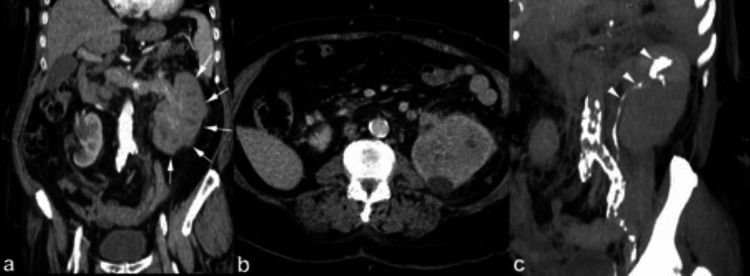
The contrast-enhanced CT (CECT) images during the arterial (a), nephrographic (b), and urographic (c) phases demonstrate the faceless kidney, which appears enlarged and displays a high density of soft tissue. Furthermore, the enhancement of the renal vein is modest due to tumoral invasion accompanied by indications of thrombosis. Large infiltrating transitional cell carcinomas (TCCs), as opposed to renal cell carcinomas (RCC), do not alter the kidney's inherent contour. Additionally, obliteration and amputation of the pyelocaliceal system are noted, which serve as indications of substantial pathological alterations occurring within the renal structure. This figure is a republished image, permission was obtained from the original publisher, https://epos.myesr.org/poster/esr/ecr2018/C-2802
